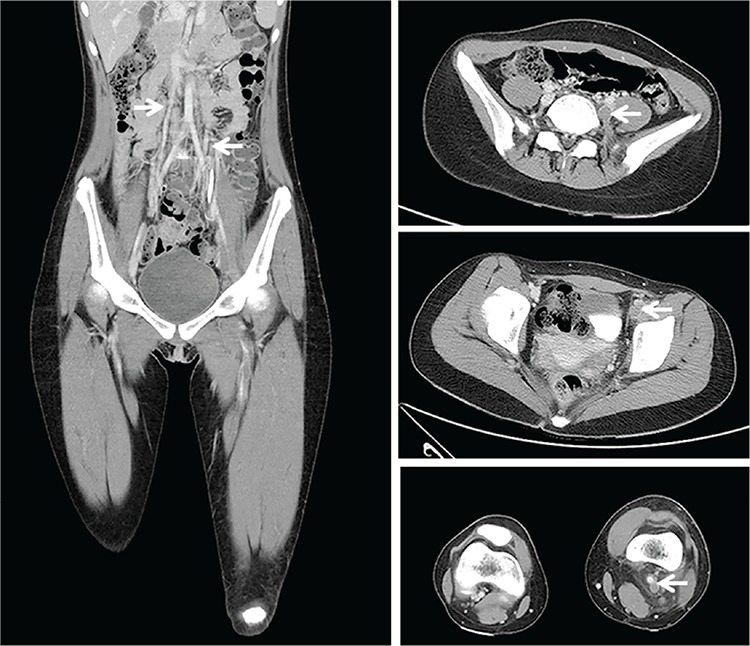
Left panel: Contrast-enhanced computed tomography image demonstrating duplicated inferior vena cava (whitish arrow). Right panel, from top to bottom: Arrow indicates thrombosis found in engorged left iliac vein, femoral vein, and popliteal vein. 254x190 mm (72x72 DPI).
